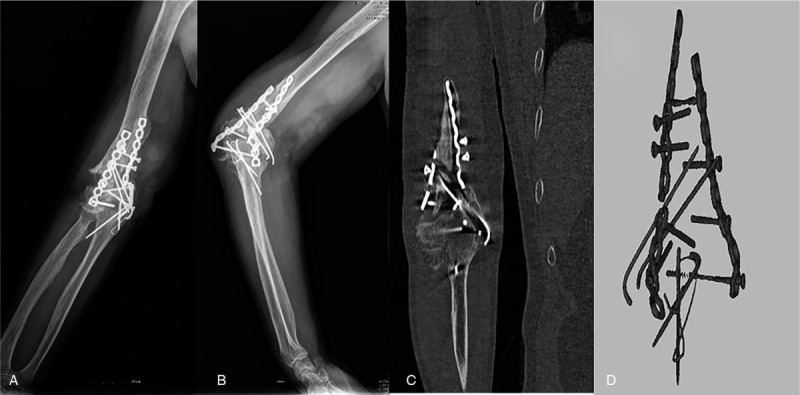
Preoperative image of a 60-year-old male patient diagnosed as fixation failure and nonunion after internal fixation of comminuted distal humerus fracture. (A) Anteroposterior plain radiograph of the right elbow joint. (B) Lateral plain radiographs of the right elbow joint. (C) Computed tomography image of the right elbow joint. (D) Reconstruction of the fractured plate by Mimics.
